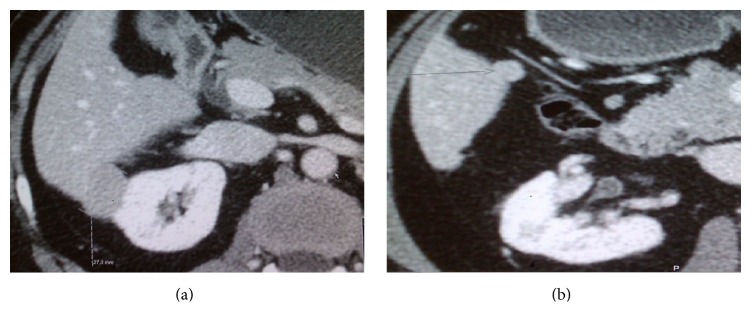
CT with intravenous contrast. Two lesions detected. First lesion adjacent to lateral part of right kidney, largest diameter 2.7 cm (a). Second lesion close to duodenum, largest diameter 1.4 cm (b).
